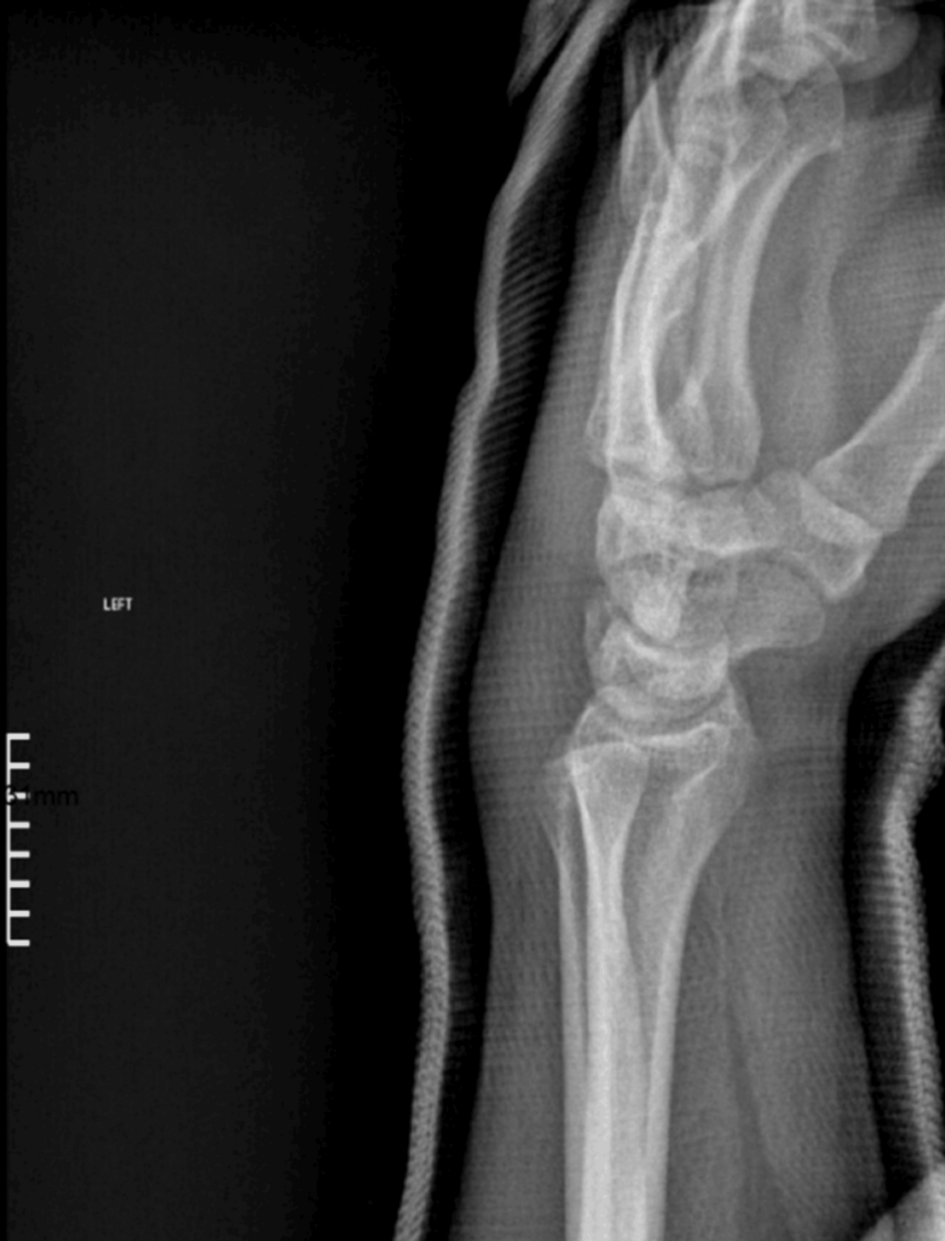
Post-reduction lateral X-ray view of the carpometacarpal joints
